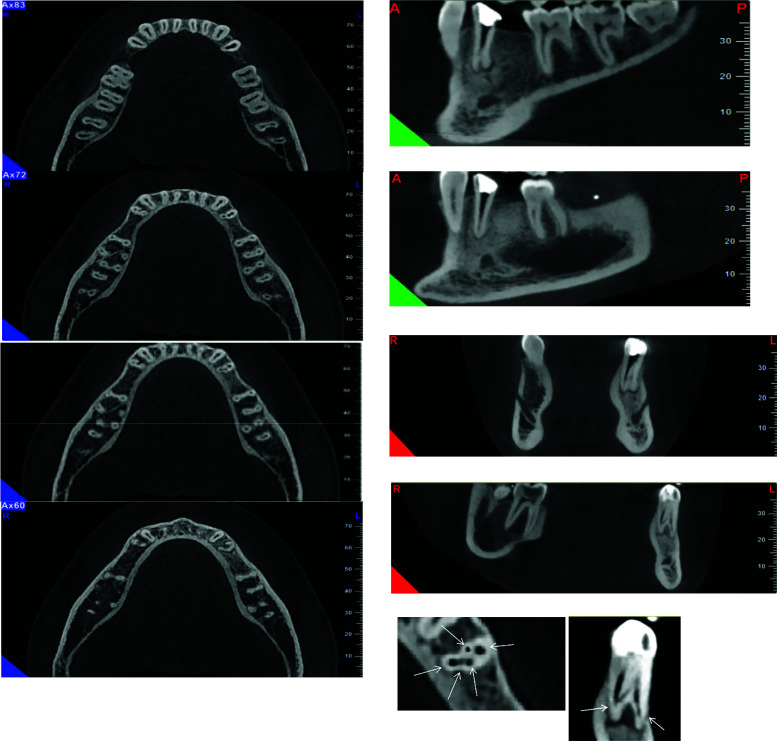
CBCT (Cone-beam Computed Tomography) images showing the roots from different planes
